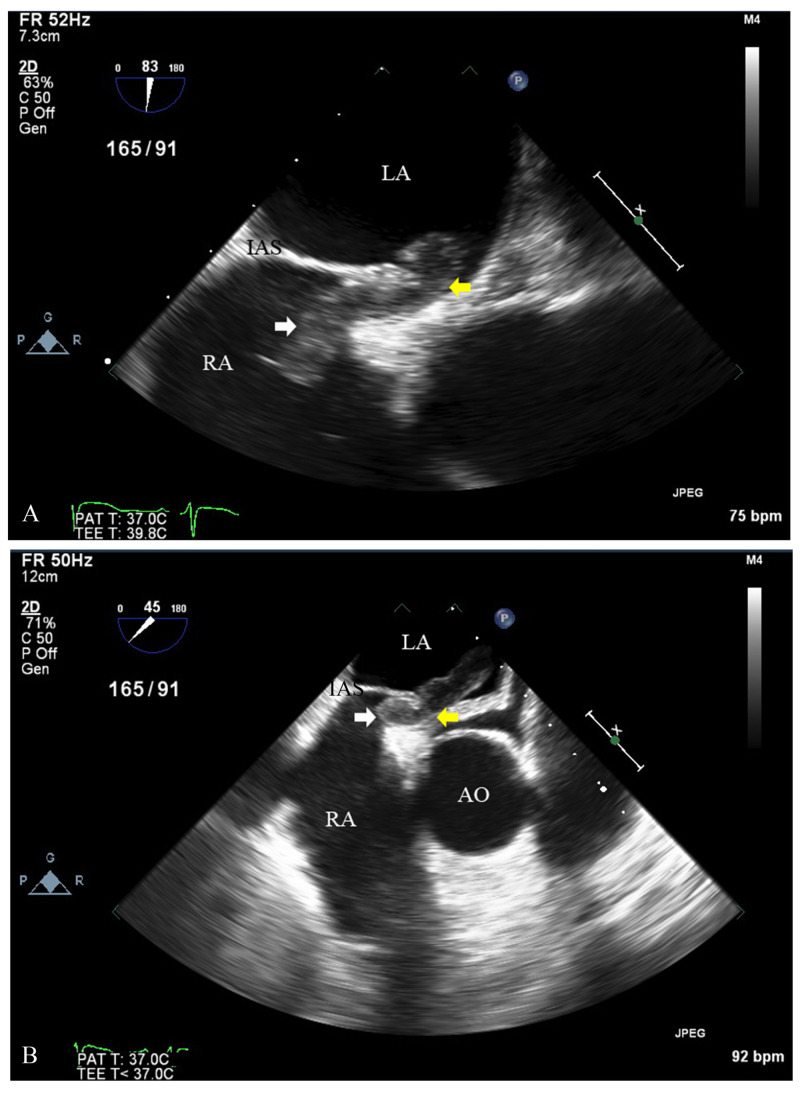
(A, B) Transesophageal echocardiogram showing a short-axis view of the aorta with thrombus (white arrow) migrating from right atrium to left atrium through patent foramen ovale (yellow arrow). RA, right atrium; LA, left atrium; AO, aorta; IAS, interarterial septum.
